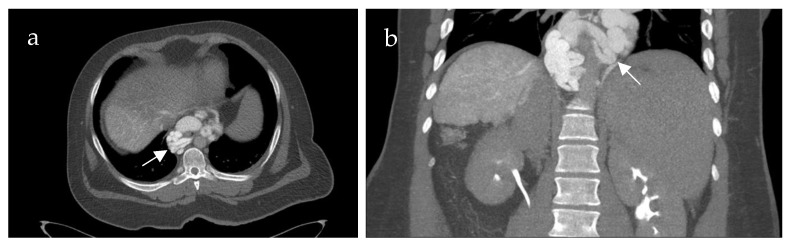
Computed tomography mesenterico-portography of a 24-year-old male patient with portal hypertension and portal vein thrombosis involving the confluence. Axial (a) and coronal (b) image after contrast injection into the superior mesenteric artery shows large contrasted periesophageal varices (white arrow) fed by the mesenteric system.
